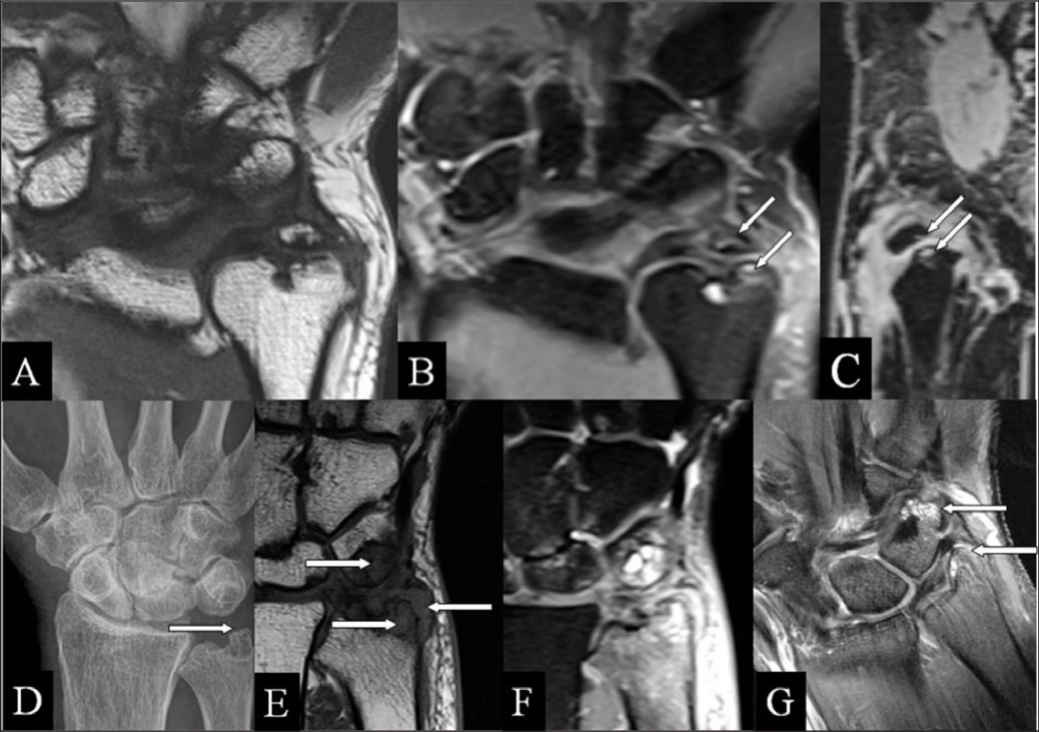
Ulnar (intra)styloid and stylotriquetral abutment. (A, E) Coronal SE T1-WI; (B, F) Coronal SE PD-WI FS; (C) Sagittal 3D-GRE; (D) PA plain radiograph; and (G) Coronal SE T1-WI FS with gadolinium. (A–C) Neoarticulation in the center of the ulnar styloid process, surrounding marrow oedema, (B) and juxta-articular cysts (arrows) (B, C). (D–F) Stylotriquetral abutment with flattening of the tip of the styloid process (D), bone marrow oedema and synovitis (E, F), and contrast enhancement of the marrow oedema and the synovitis (G).
